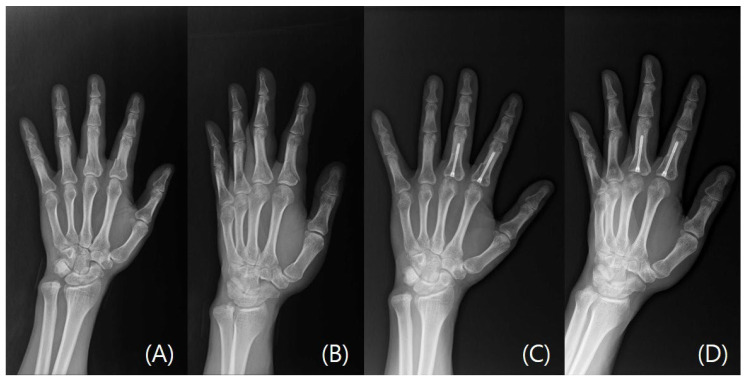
A case of left second and third digit short oblique proximal phalangeal fractures. (A) Preoperative plain radiograph Posteroanterior view. (B) Preoperative plain radiograph oblique view. (C) Postoperative plain radiograph Posteroanterior view taken at last follow-up visit. (D) Postoperative plain radiograph oblique view taken at last follow-up visit.
